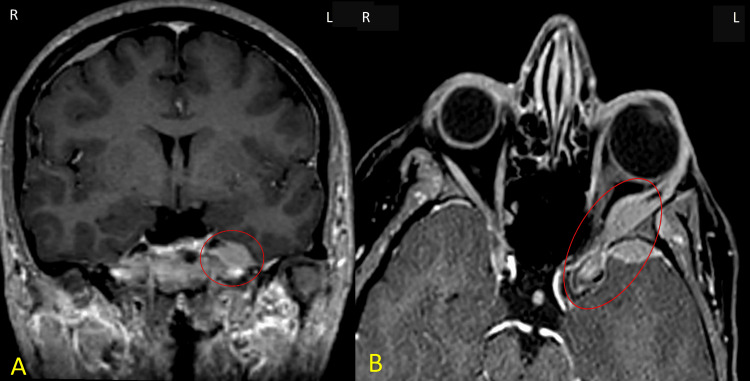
MRI of CNS and Orbital Involvement in Multiple Myeloma (A) Axial post-contrast T1-weighted MRI showing a homogeneously enhancing dural-based lesion (circle) adjacent to the left cavernous sinus and anterior temporal lobe. (B) Coronal post-contrast T1-weighted orbital MRI showing an intraorbital lesion (circle) causing mass effect on the distal aspect of the left optic nerve. MRI, magnetic resonance imaging; CNS, central nervous system
